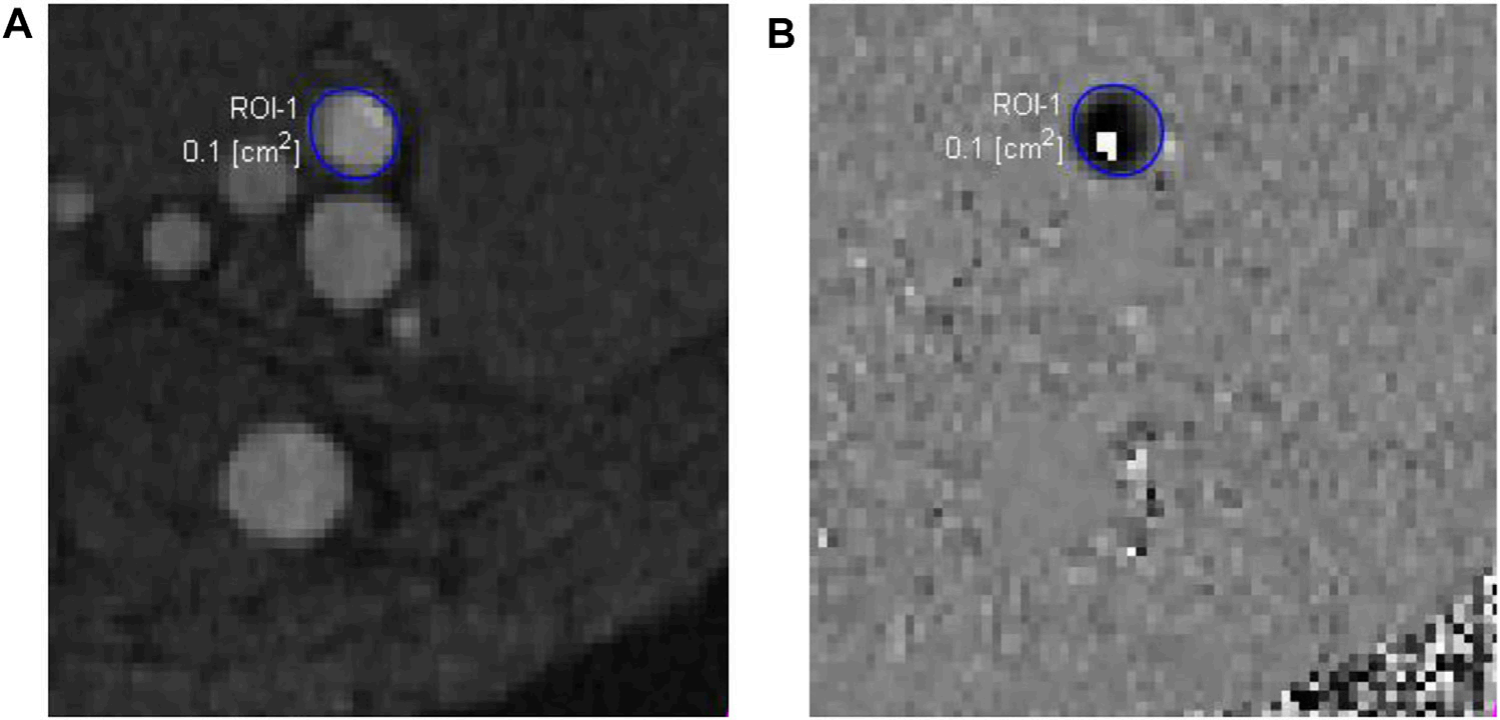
Phase-contrast magnetic resonance images of an arteriovenous fistula. The magnitude image (A) is used to draw a region of interest (ROI-1) for the proximal artery (enclosed by the blue circle), which may have very high flow velocity. In this case, the velocity encoding value, 250 cm/s, was not big enough, causing a phase-wrap artifact shown by the white pixels within the ROI-1 in the phase image (B).
